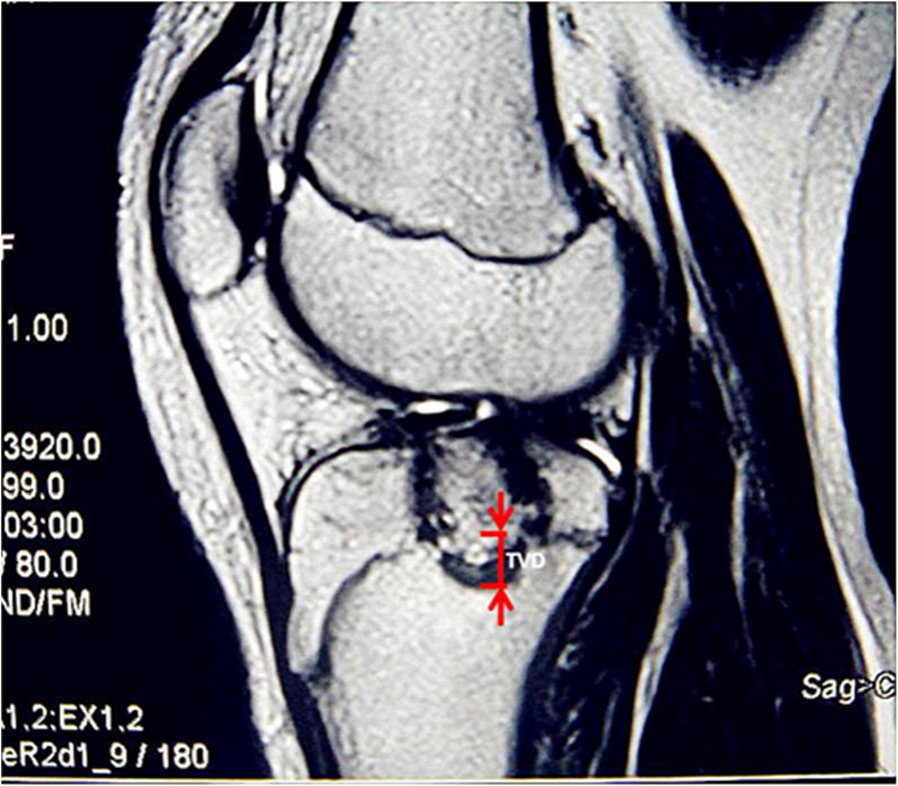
TVD, the vertical dimension between the edge of lesion and epiphyseal line, was from the lower edge of the lesion to the epiphyseal line in the vertical plane of the sagittal or crown MRI plane. TVD is a negative data when the lesion is crossing the epiphyseal line
